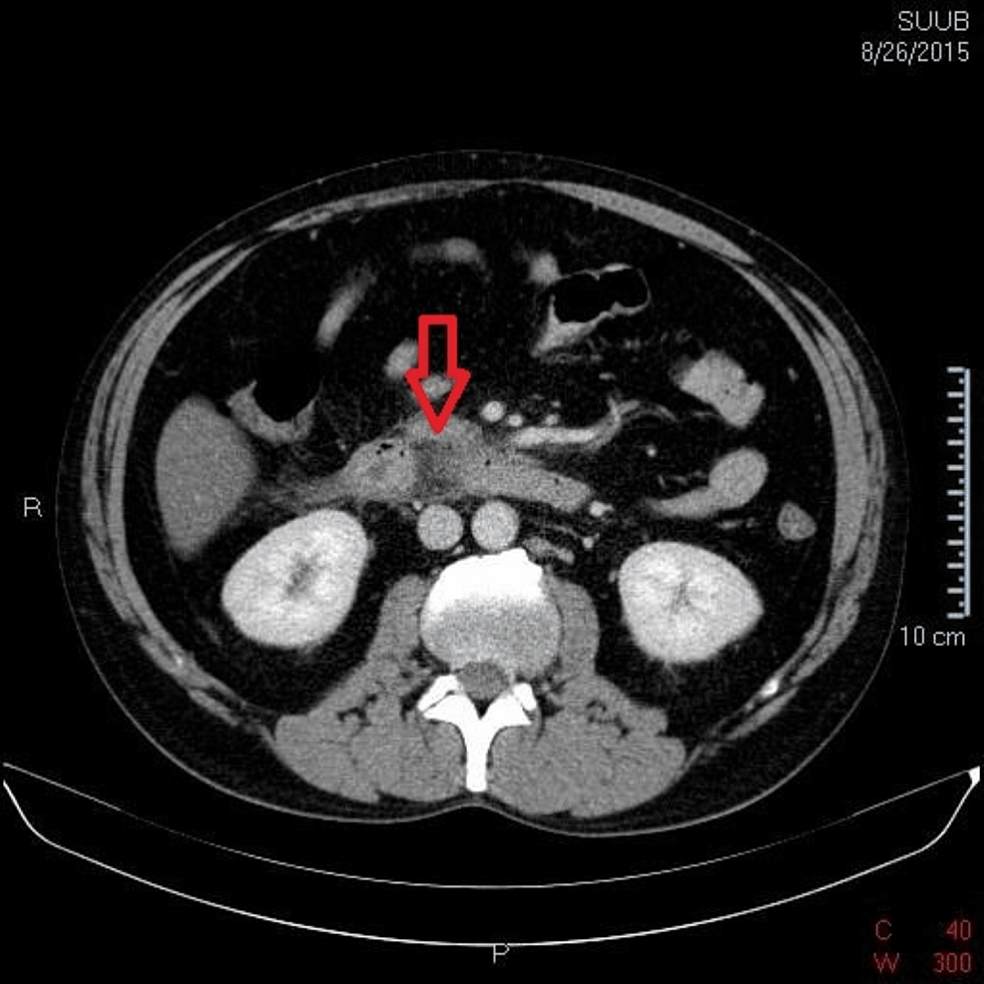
Typical CT-scan alterations in acute pancreatitis
